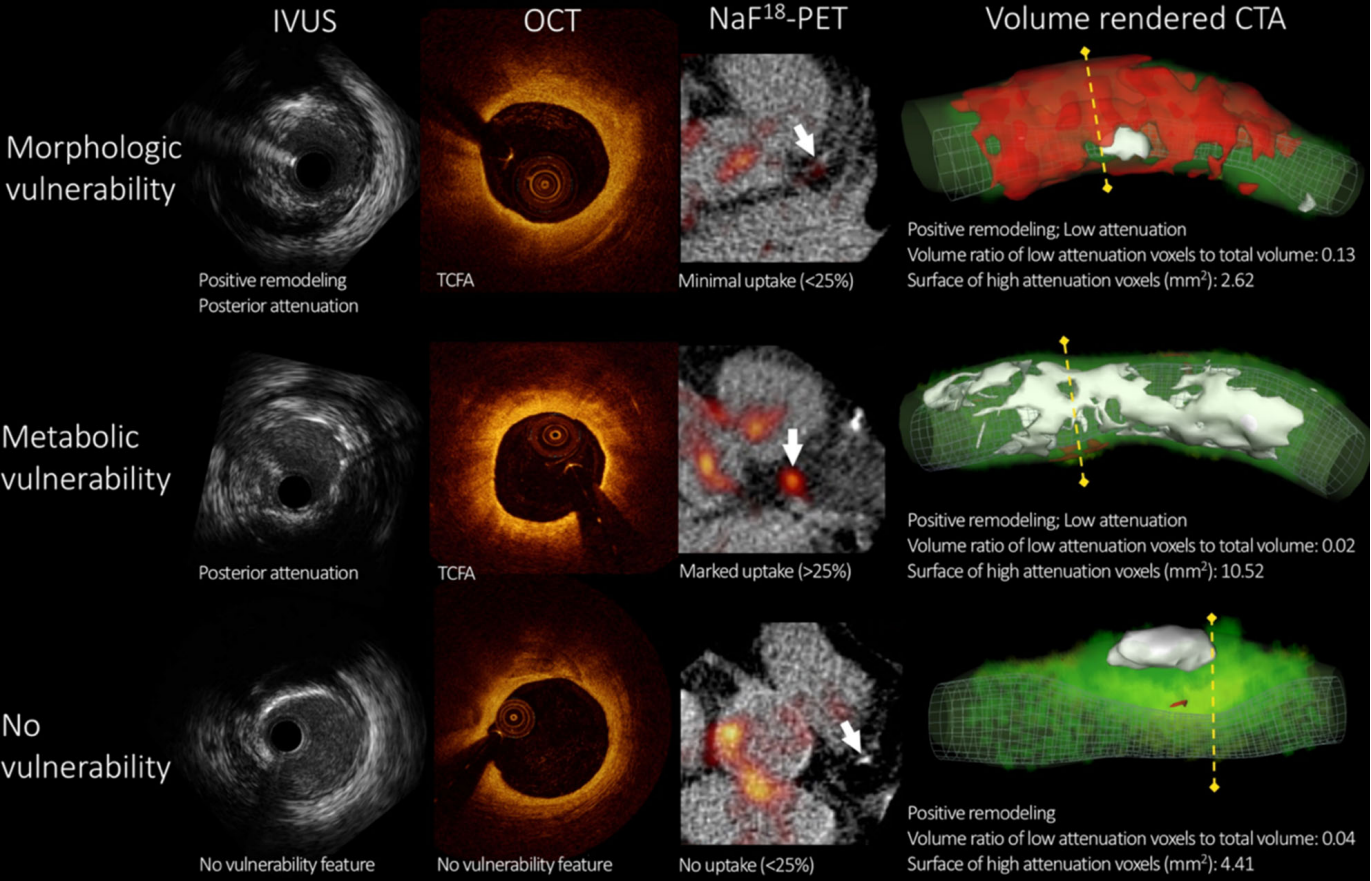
Intravascular ultrasound, optical coherence tomography, 18F-sodium fluoride-PET, and volume-rendered coronary computed tomography angiography images of three representative coronary lesions to assess plaque vulnerability. Morphologic vulnerability was defined as the presence of positive remodeling and posterior attenuation on intravascular ultrasound (IVUS) and the presence of thin-cap fibroatheroma (TCFA) or intraplaque microvessels or macrophage infiltration on optical coherence tomography (OCT). Metabolic vulnerability was defined as any plaque showing > 25% uptake on 18F-sodium fluoride-PET (NaF^18^-PET) images. *Upper row* depicts a plaque showing morphologic characteristics of vulnerability. *Middle row* shows a lesion with metabolic characteristics of vulnerability. *Bottom row* displays a plaque showing neither morphologic nor metabolic characteristics of vulnerability. Image courtesy of M. Kolossvary. CTA, computed tomography angiography
